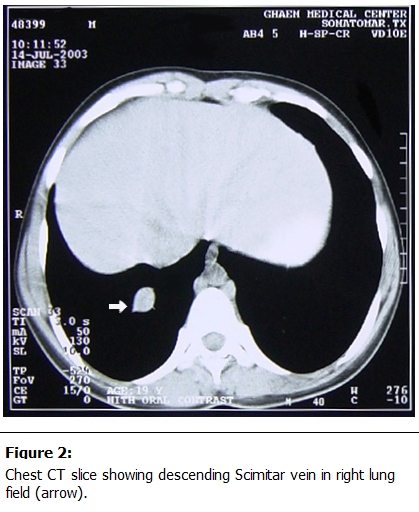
Chest CT slice showing descending Scimitar vein in right lung field (arrow).
